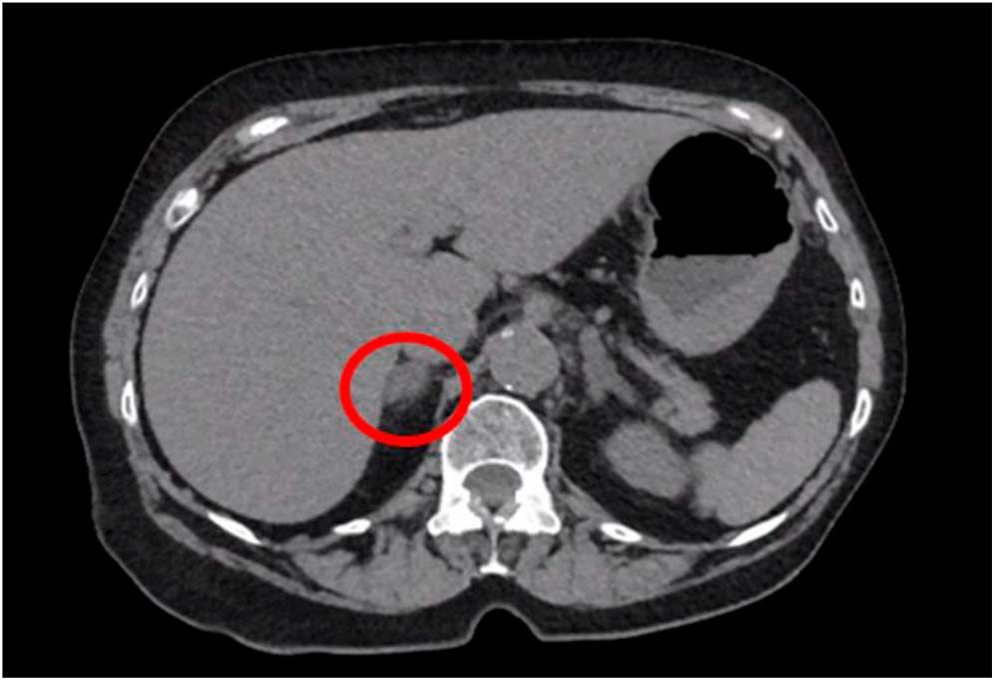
CT abdomen, pre-contrast phase.
